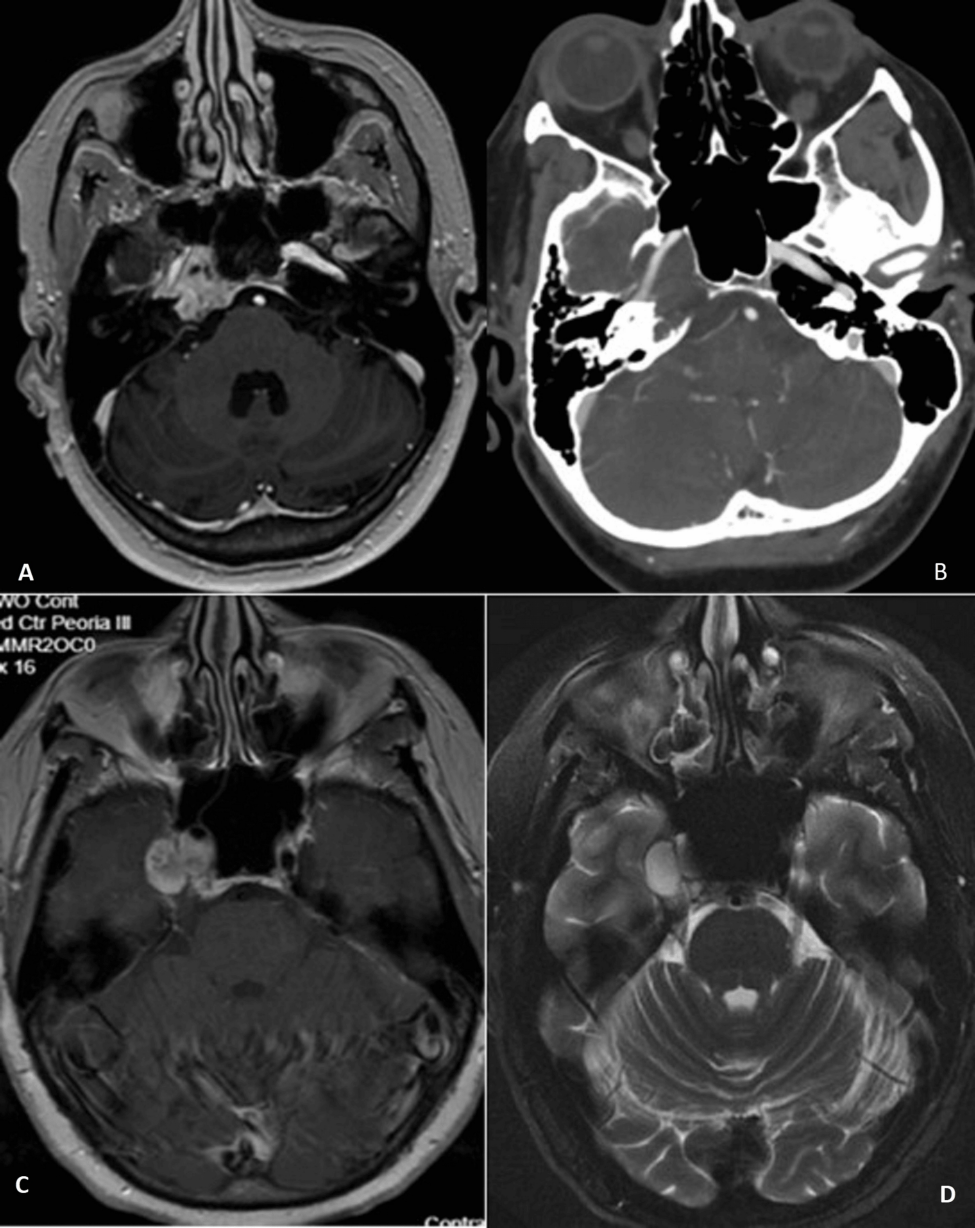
Preoperative imaging (A) T1 post-contrast sequence of the petroclival junction enhancing lesion, (B) Computed tomography angiography of the lytic lesion of the petrosal bone with overlying internal carotid artery (ICA) in the carotid canal, (C) overlying cystic component intradurally within the cavernous sinus on T1 post-contrast, and (D) T2 also showing this same cavernous sinus lesion which was targeted for biopsy.
